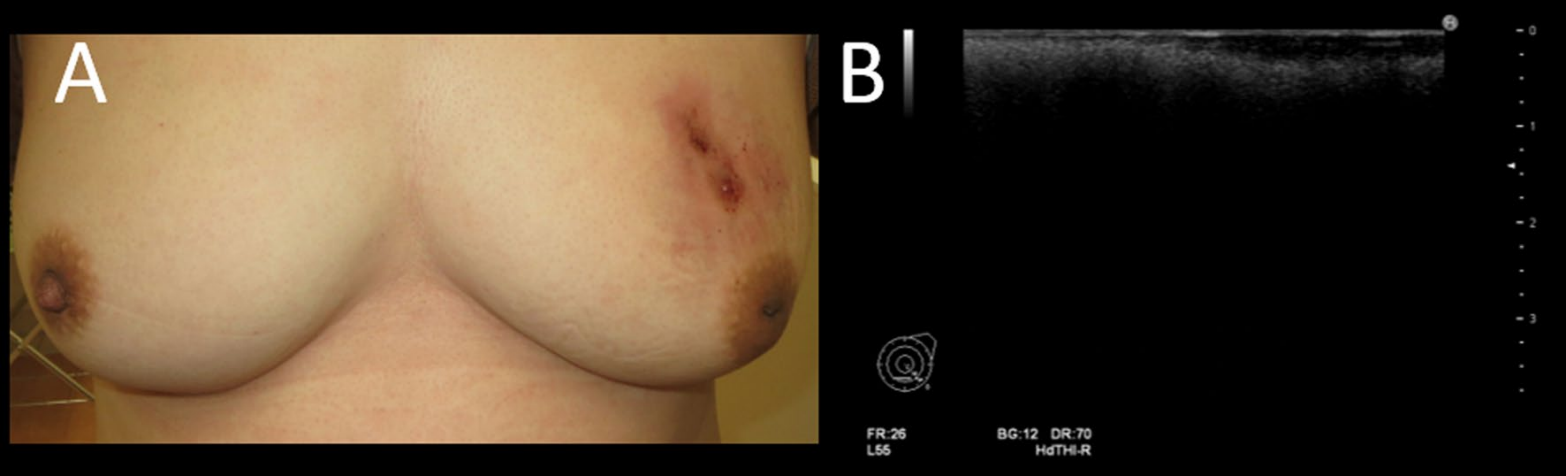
Skin findings and ultrasound images at initial examination. A Skin of the left breast was partially destructed and there was leakage of pus and serous fluid. B It was difficult to observe the depth due to strong backward echo caused by foreign body
